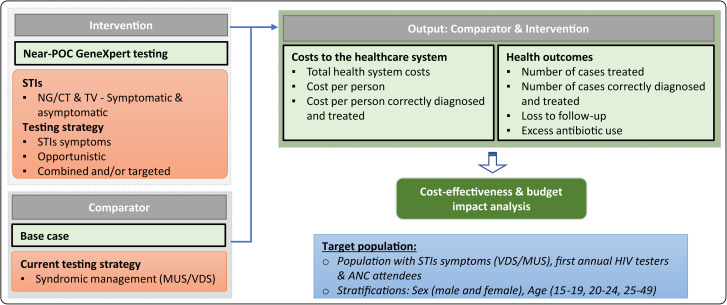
The modelling structure and approach*. *Abbreviations: STIs = sexual transmitted infections, ANC = antenatal care; MUS = male urethritis syndrome, VDS = vaginal discharge syndrome, HIV = human immunodeficiency virus; NG = Neisseria gonorrhoea; CT = Chlamydia trachomatis; TV = Trichomonas vaginalis; POC = point-of-care.
